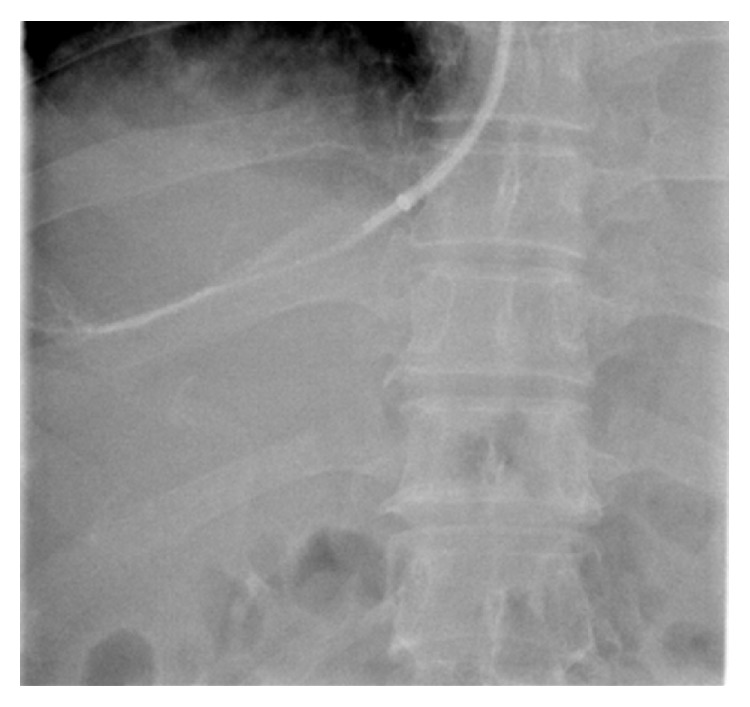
Hepatic venous blood samples were taken under guidance of X-rays.
